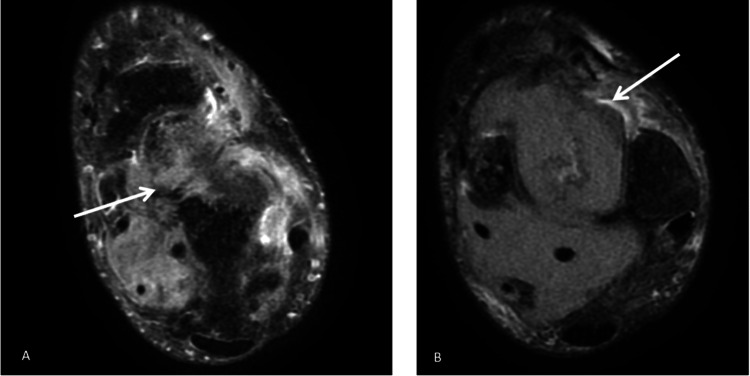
Axial MRI of the left ankle, obtained with T2-weighted sequences following contrast administration, reveals abnormal signal intensity and soft tissue involvement surrounding the distal tibia, consistent with an aggressive lesion. (A) demonstrates involvement around the talus, while (B) highlights involvement in the soft tissue surrounding the talus. The white arrows indicate the area of the lesion. MRI: magnetic resonance imaging
